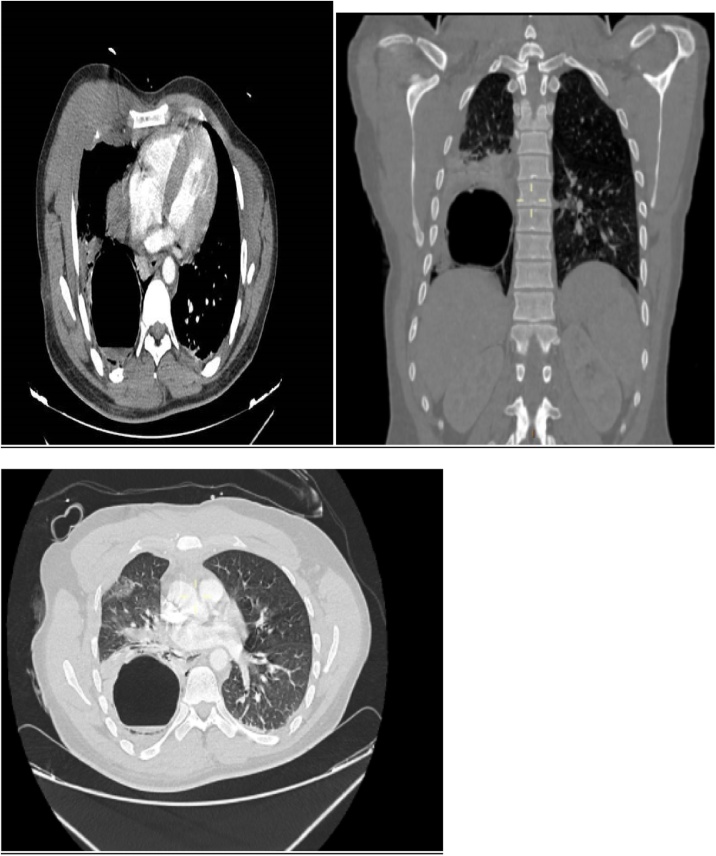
CT SCAN showing well limited rounded formation of regular contours containing a hydro-aeric level related to a ruptured hydatid cyst.
